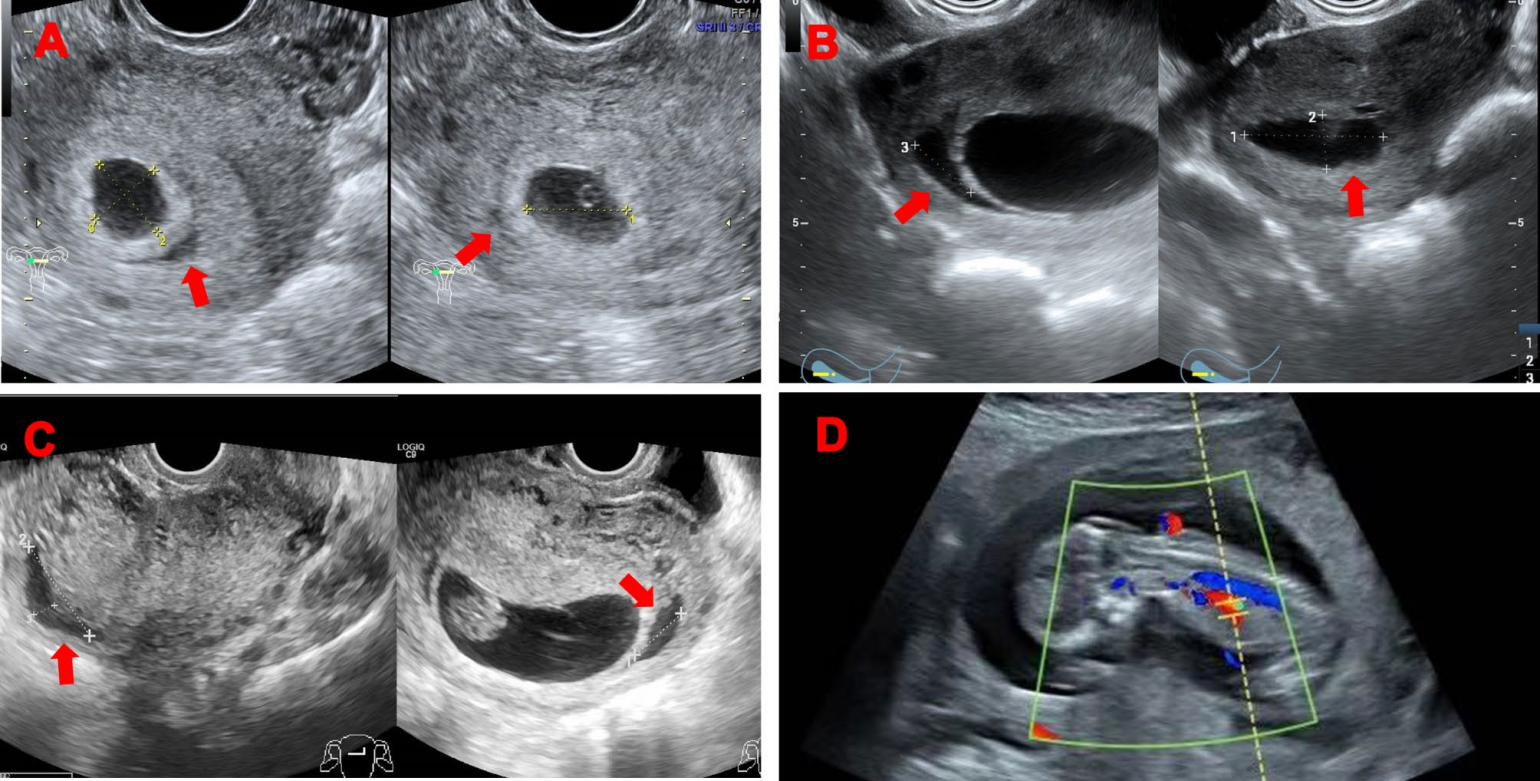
Ultrasound images of a single patient at different gestational ages in the subchorionic hematoma group. A: At 5 weeks and 4 days of gestation with subchorionic hematoma measuring 1.85*1.64*1.42 cm^3^. B: At 8 weeks and 1 days of gestation with subchorionic hematoma measuring 3.11*1.23*1.25 cm^3^. C: At 10 weeks and 1 days of gestation with subchorionic hematoma measuring 1.74*3.00*0.62 cm^3^. D: The subchorionic hematoma disappeared at 13 weeks and 1 days of gestation. *Arrows* indicate subchorionic hematoma. The hematoma size used for analysis in this study was the initial size of the hematoma measured at 5–10 weeks
